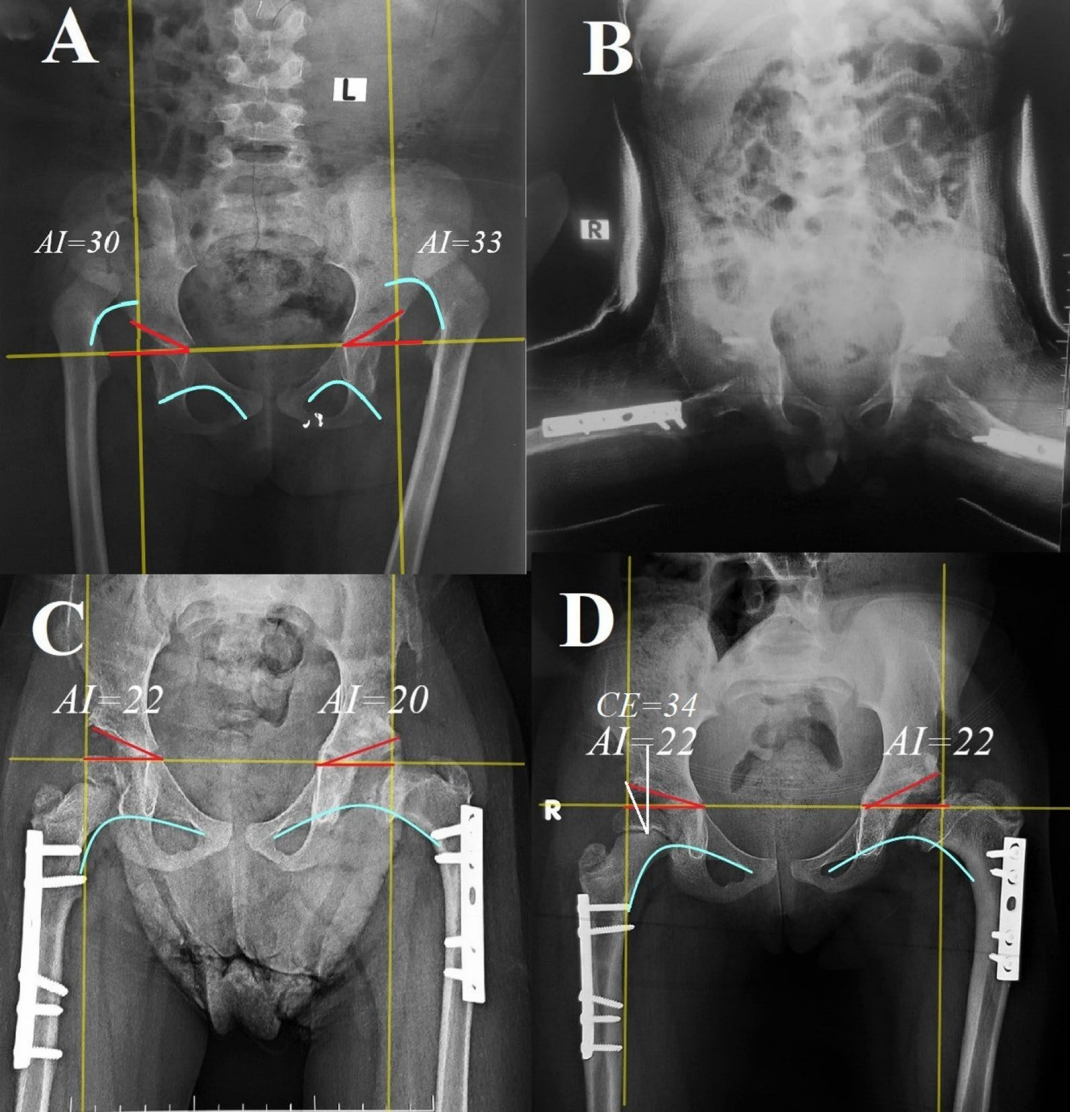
Female child, 70 months, presented with bilateral DDH, Tönnis grade IV. A preoperative. B Immediate postoperative. C After 6 months of follow-up. D After 18 months of follow-up. Full union of the osteotomies and the radiographic result was Severin’s grade I on the right side and grade III on the left side. The left hip shows Kalamchi and MacEwen AVN grade IV
